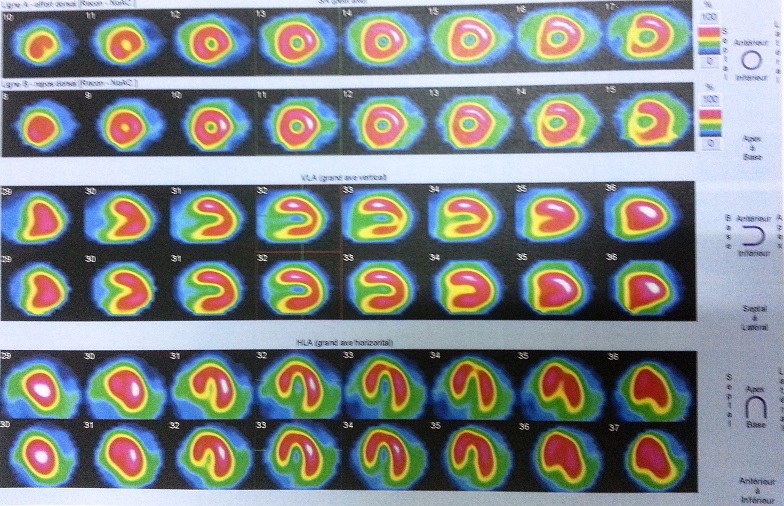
High-resolution computed tomography scan of the chest showing peribronchial and bilateral hilar lymphadenopathy (arrows)
